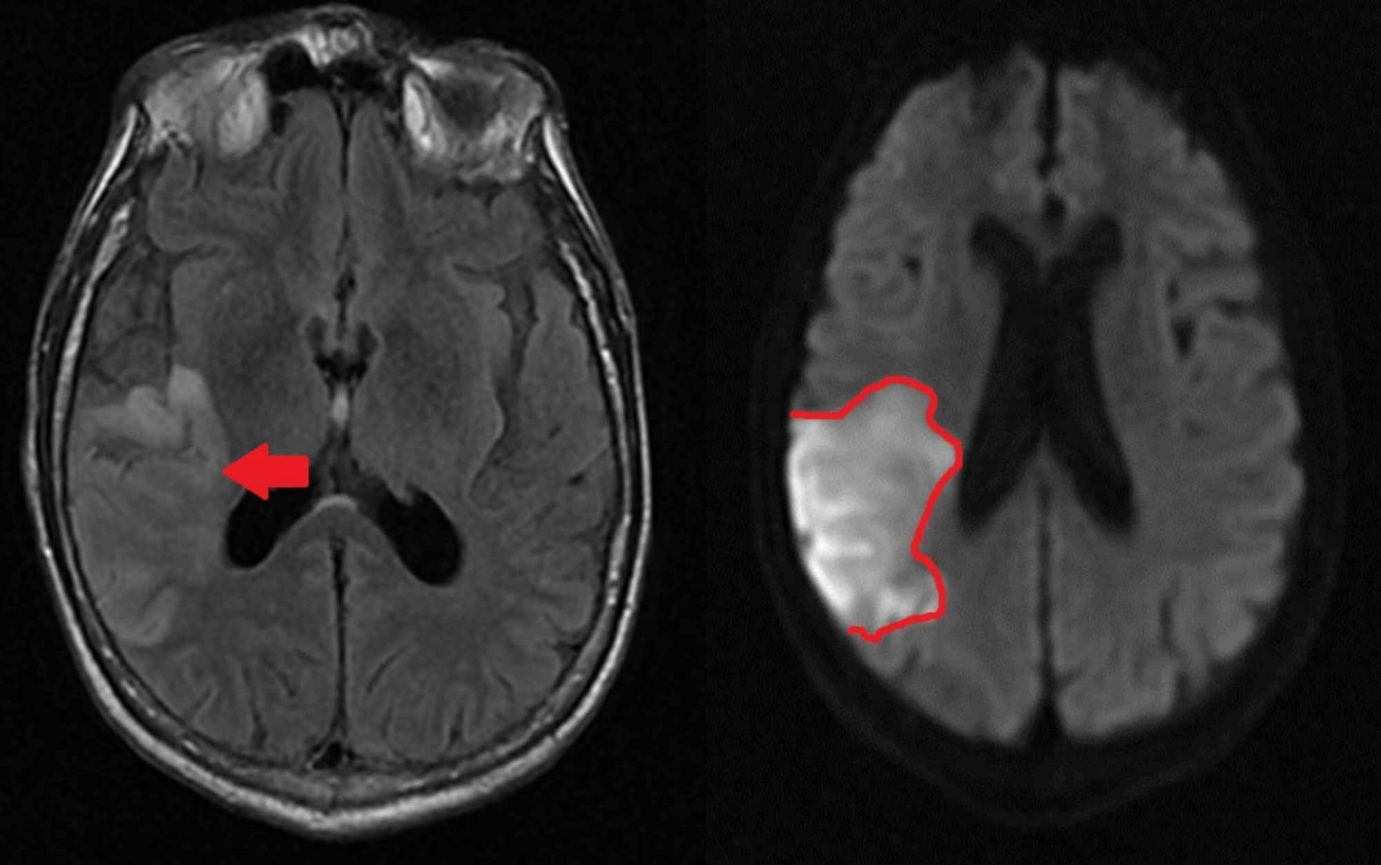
Large right middle cerebral artery region infarct visualized with MRI *Left: *Axial view MRI with fluid-attenuated inversion recovery (FLAIR). Area of infarct is indicated by an arrow.* Right:* MRI with diffusion-weighted imaging (DWI), infarcted area has been outlined
